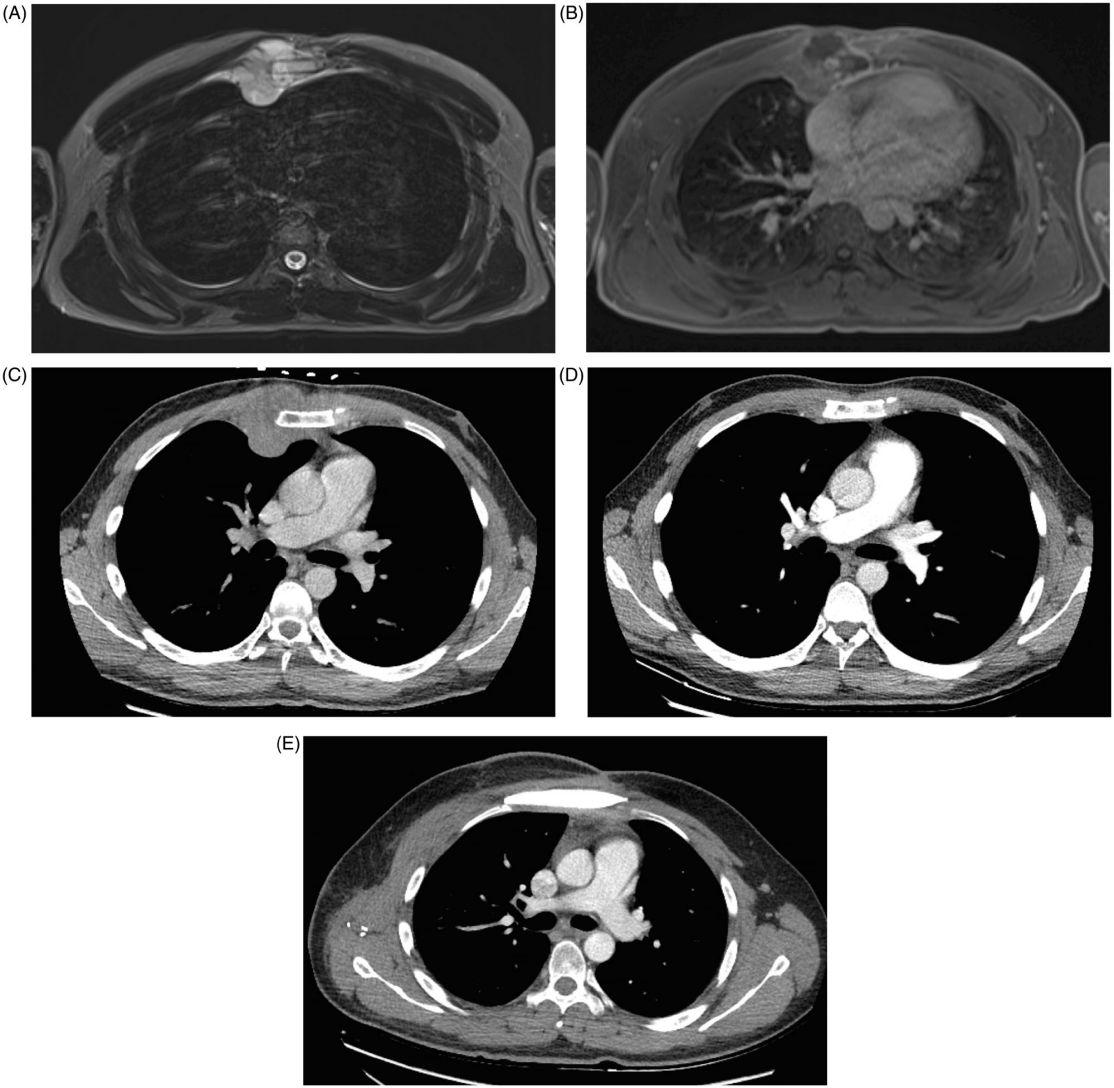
Axial T2-weighted MR-image (A) shows an area of abnormal signal intensity (bone involvement) in the sternum with associated right parasternal soft tissue component. The soft tissue mass has a lobulated morphology, is well defined and has a high signal intensity. Axial T1-weighted images after intravenous gadolinium contrast administration (B) show a heterogeneous lesion with peripheral enhancement and central areas of low signal intensity, corresponding to areas of necrosis. Axial CT-images at the time of diagnosis (C) show an abnormal density in the sternum with large soft tissue component. Follow-up CT after neoadjuvant chemotherapy (D) shows prominent shrinkage of the mass. Follow-up CT after surgery (E) shows normal postoperative findings after partial sternal resection and reconstruction.
